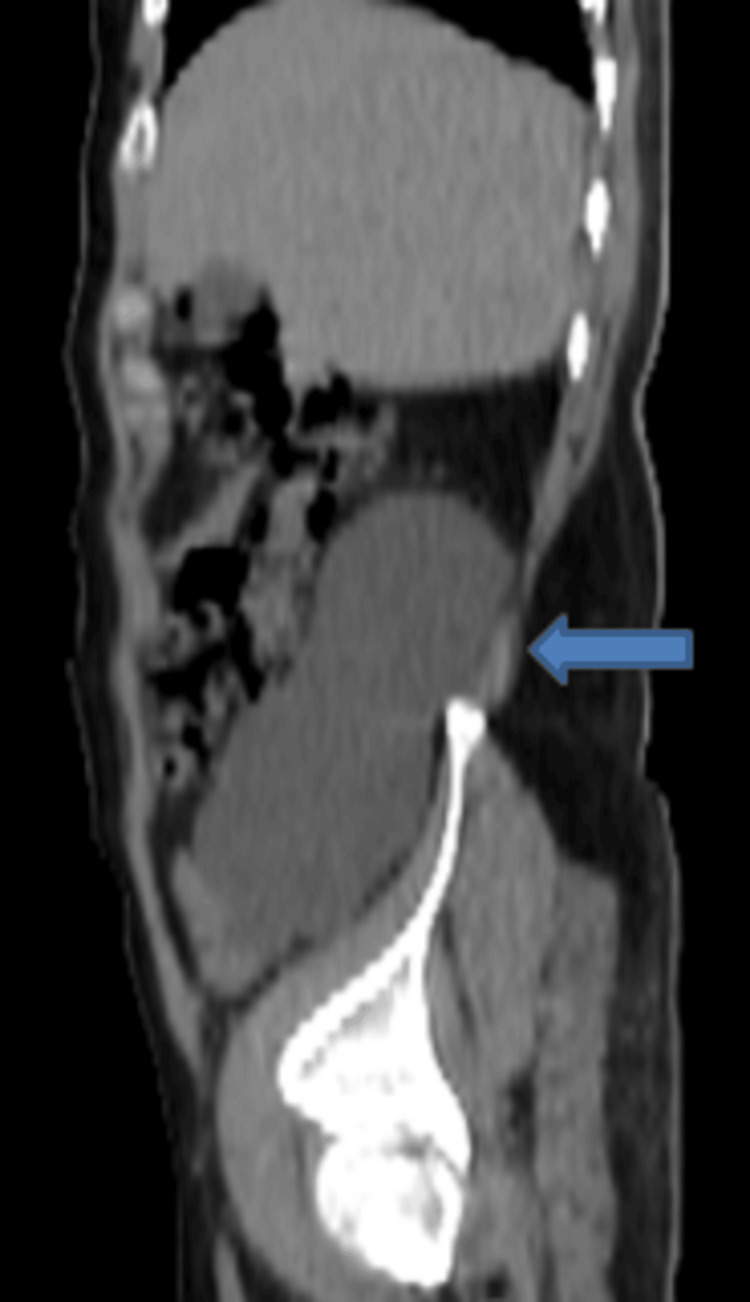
Non-contrast CT of the abdomen (sagittal view) showing an encapsulated fluid collection in the right lumbar region (blue arrow). The collection appears to be inseparable from the right ureter with moderate dilatation of the right collecting system.
